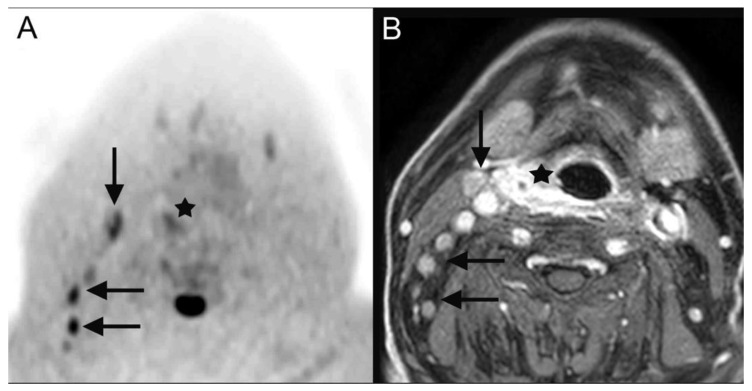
DWIBS (A) as well as contrast-enhanced fat-saturated T1-weighted MR images (B) depict the primary cancer (A,B: black star) lesion as well as the nodal metastasis (A,B: black arrows) in a 70-year-old male patient who was diagnosed with a right-sided hypopharyngeal carcinoma (cT2 cN2b cM0).
